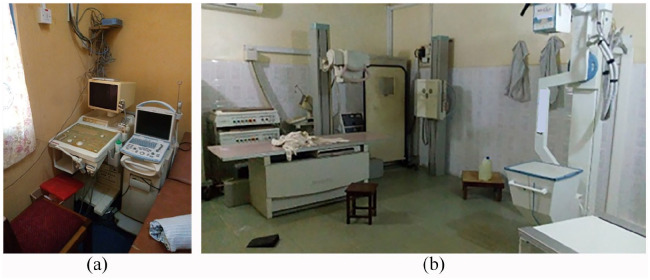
Inappropriate use of devices: (a) redundant ultrasound scanner operating
